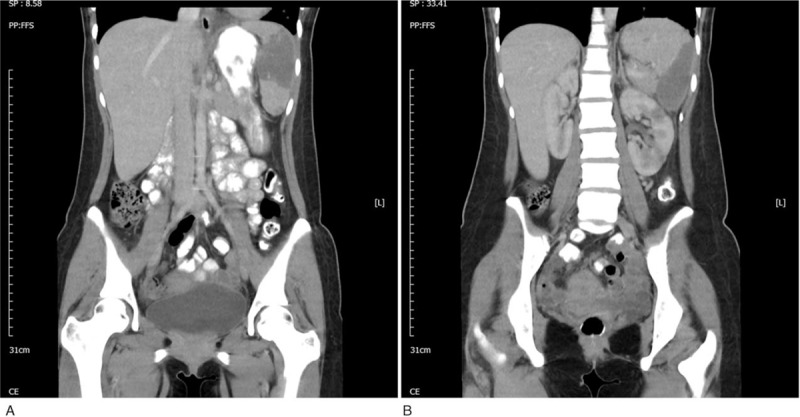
Abdominal computed tomography revealed splenic and left renal infarction.
